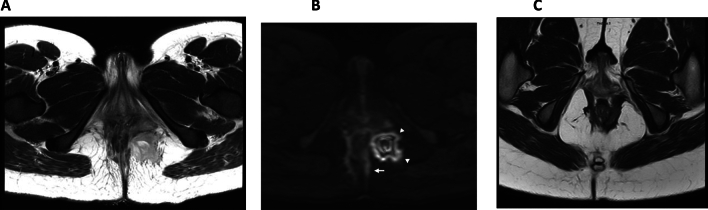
Pre- and postoperative MRI in patient with Crohn’s disease showing clinical and imaging response after surgical fistula closure. A, B Preoperative T2W- (A) and DWI- MRI, b = 1000 s/mm^2^ (B) show a transsphincteric fistula (arrow) with presence of a perianal abscess (arrowheads); C postoperative T2W MRI showing radiological resolution with absence of fistula activity and abscess (preoperative Original van Assche score of 16 points versus a postoperative Original van Assche score of 0 points)
